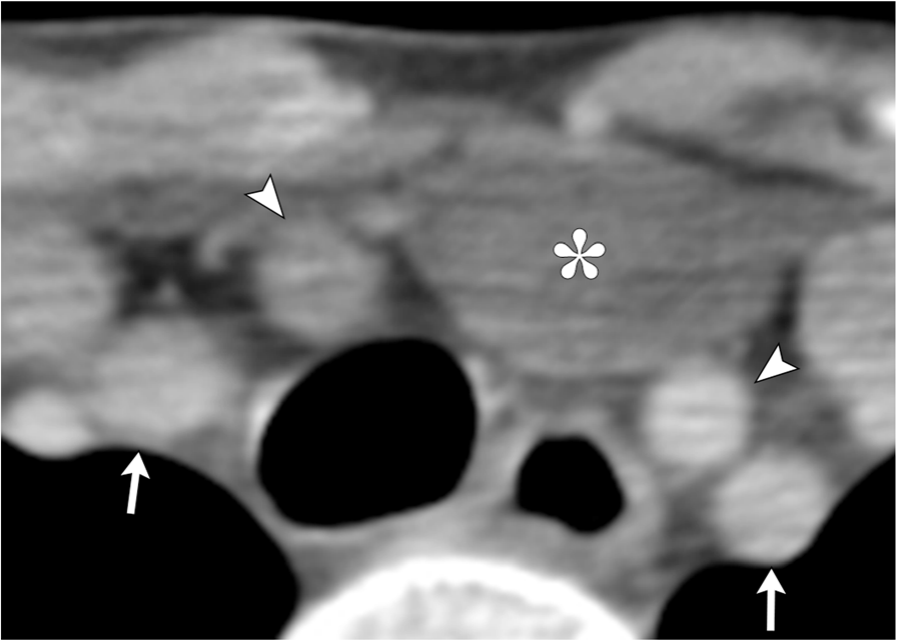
Thymic Cyst. Axial contrast-enhanced CT image demonstrates a cystic mass (asterisk) in the left neck base between the right and left common carotid arteries (arrowhead), extending into the superior mediastinum. Bilateral subclavian arteries are also visualized (arrows)
